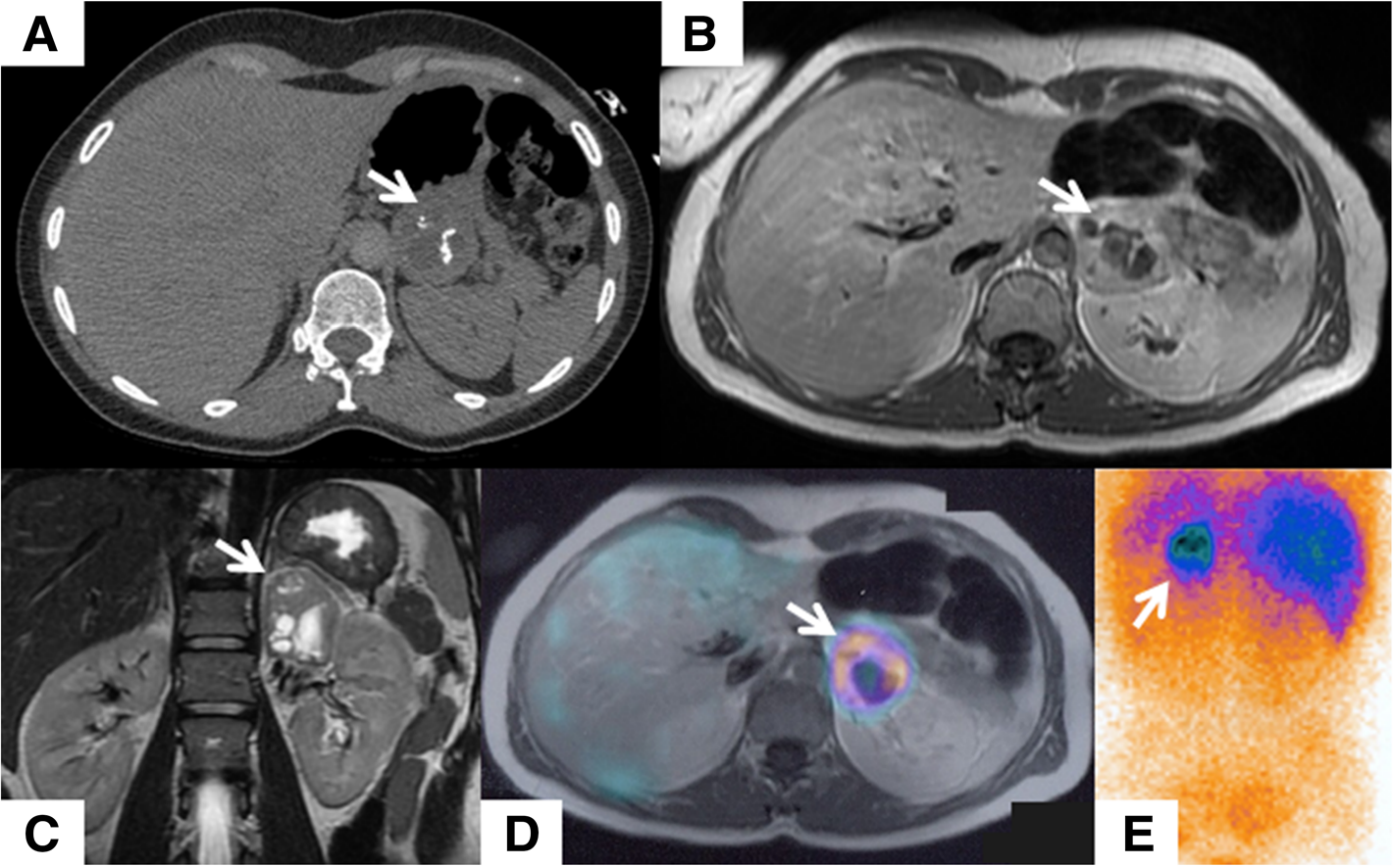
Pheochromocytoma imaging features. Pheochromocytoma mass (arrows) with heterogeneous appearance in computed tomography (A), revealing hypointense signal on T1-weighted (B) and hyperintense signal on T2-weighted (C) sequences of magnetic resonance imaging, and high uptake on ^123^I-mIBG scintigraphy (D,E).
